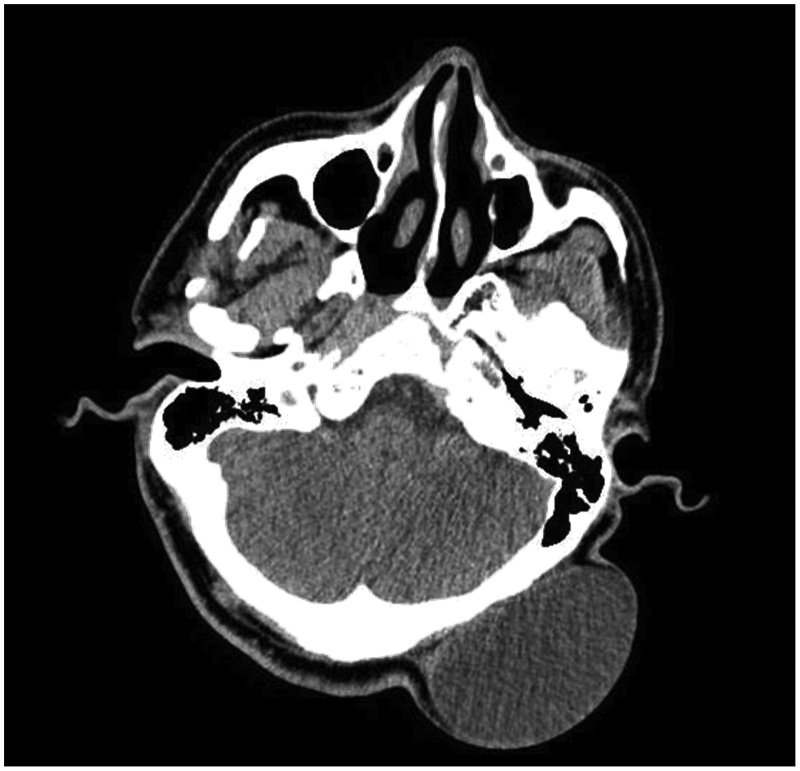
CT head showing subcutaneous extra cranial cystic lesion with no intracranial connections.
